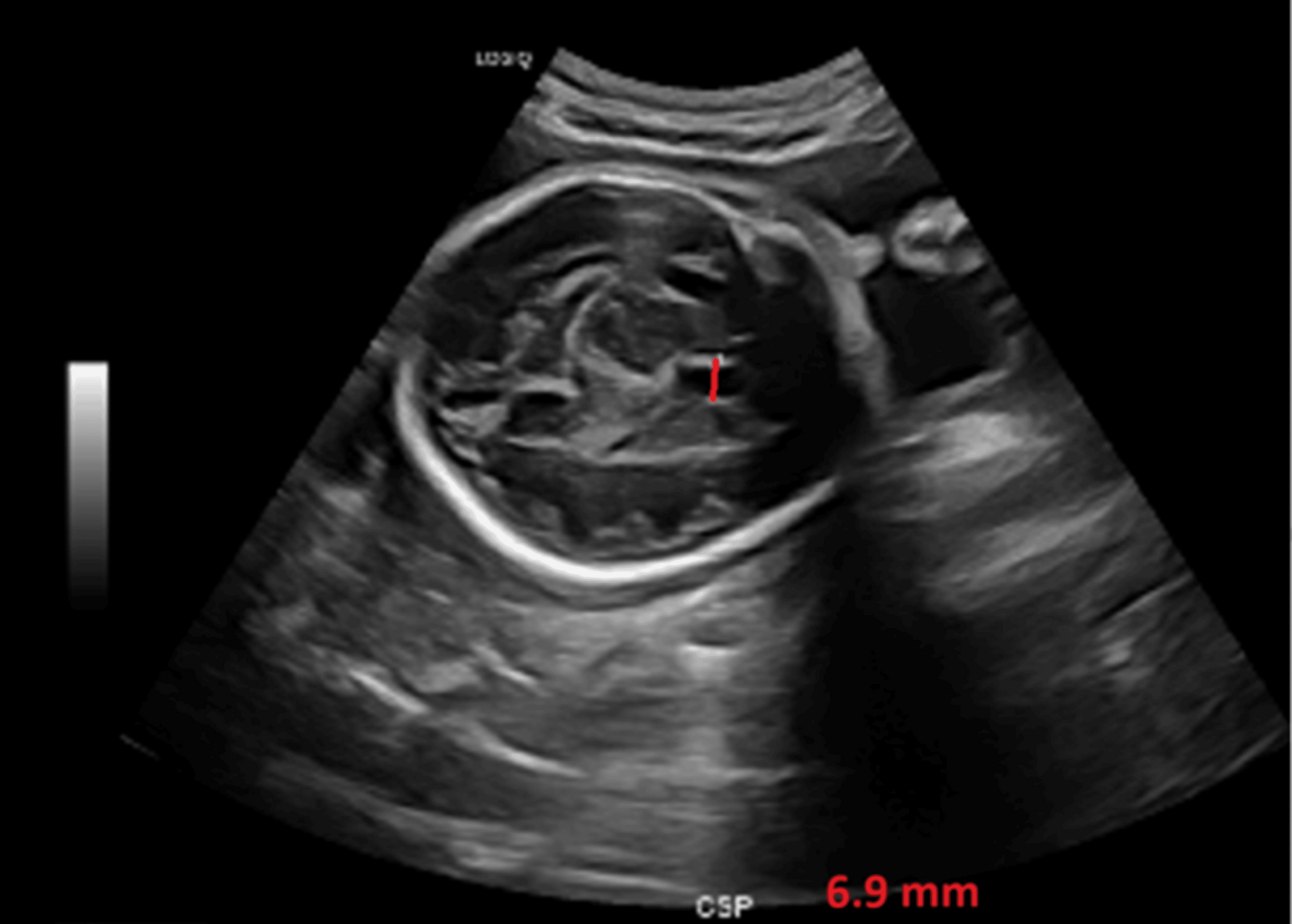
Follow-up ultrasound at 29 weeks demonstrating the CSP (red line) measuring 6.9 mm, with resolution of the previously noted enlargement CSP: cavum septum pellucidum
